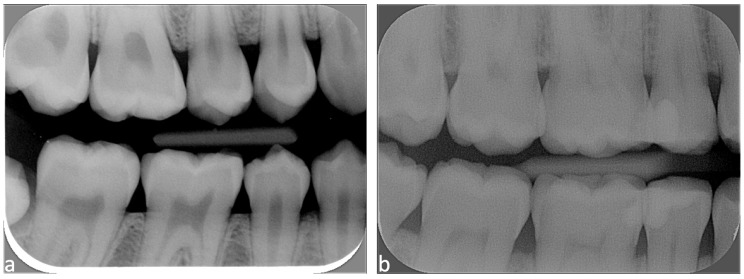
Bitewing scores presented from left to right: (a) score 11/14: good X-ray; and (b) score 4/14: unacceptable X-ray.
